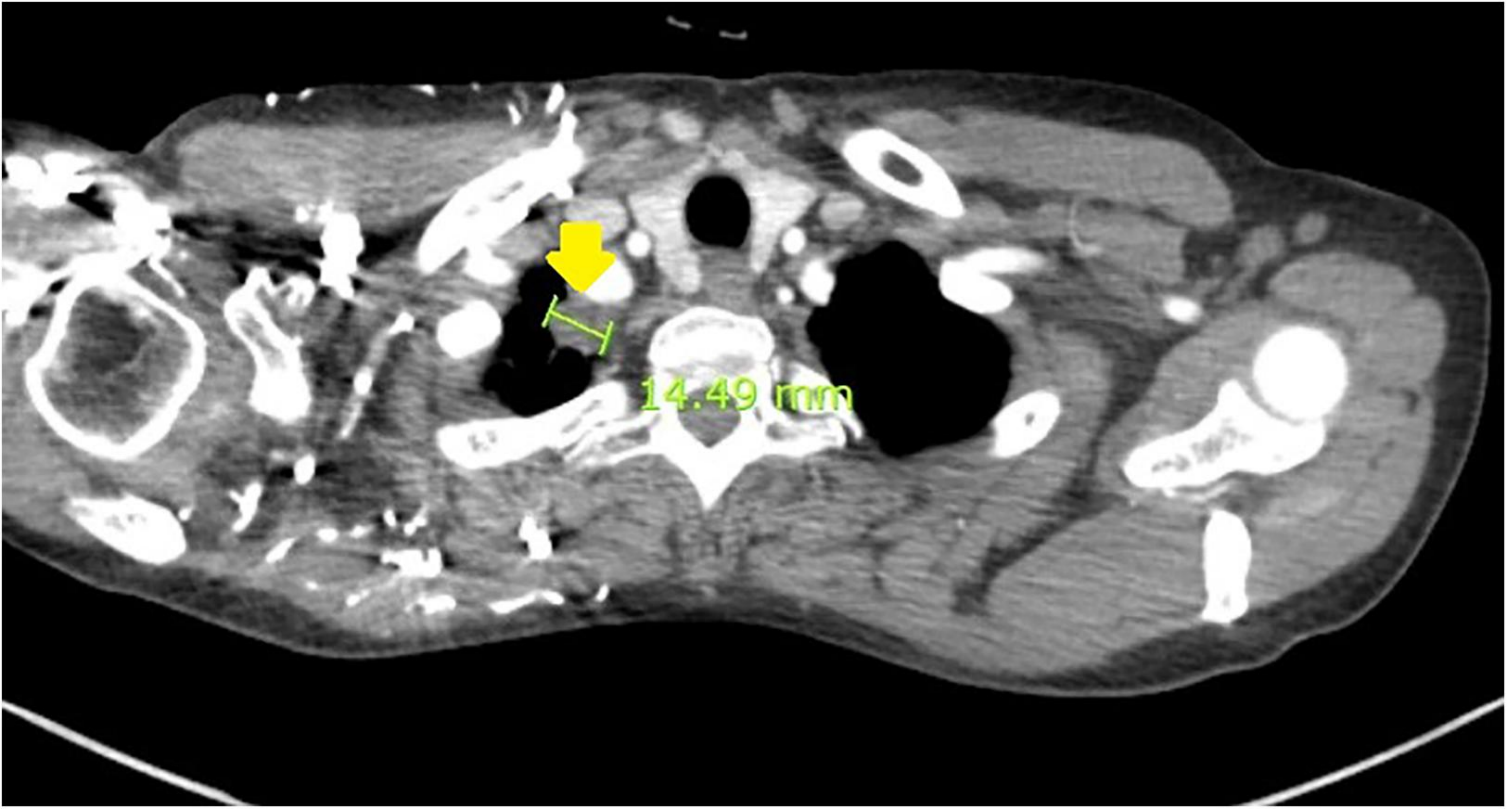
Chest CT showing an apical 1.5-cm mass (yellow arrow) and enlarged lymph nodes in the right upper lung.
